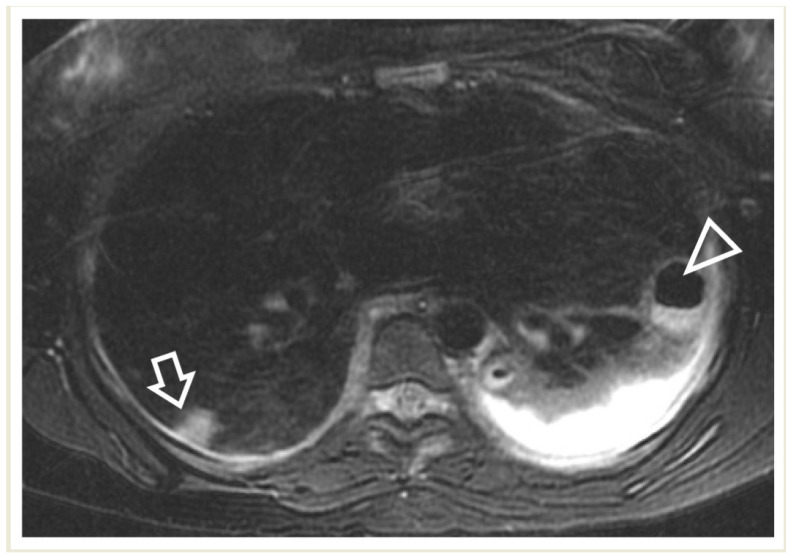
A 17-year-old girl with a pulmonary abscess following septic embolism. Lung MRI, in an axial T2-weighted image, shows areas of high signal intensity consistent with subpleural septic emboli (arrow) and pleural effusion (very high-signal-intensity area dorsally, mostly on the left side). Note the abscess at the left lung base, exhibiting a low signal and relatively thick wall, and containing an air–fluid level (arrowhead).
